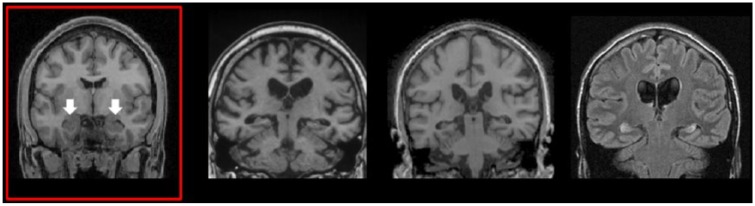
The human hippocampus. Coronal sections from magnetic resonance imaging brain scans: left panel (red box), the arrows indicate the two hippocampi of a healthy control participant. The other panels show scans of patients with bilateral hippocampal damage.
